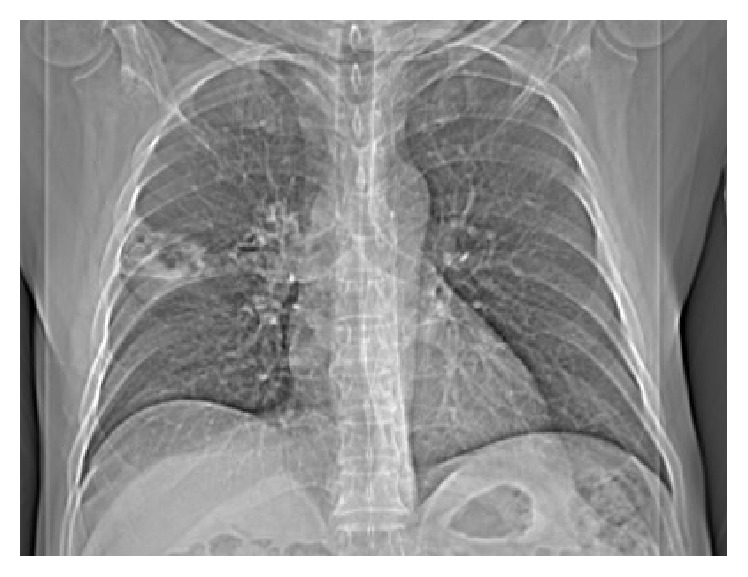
Chest computed tomographic scan at ER admission.
